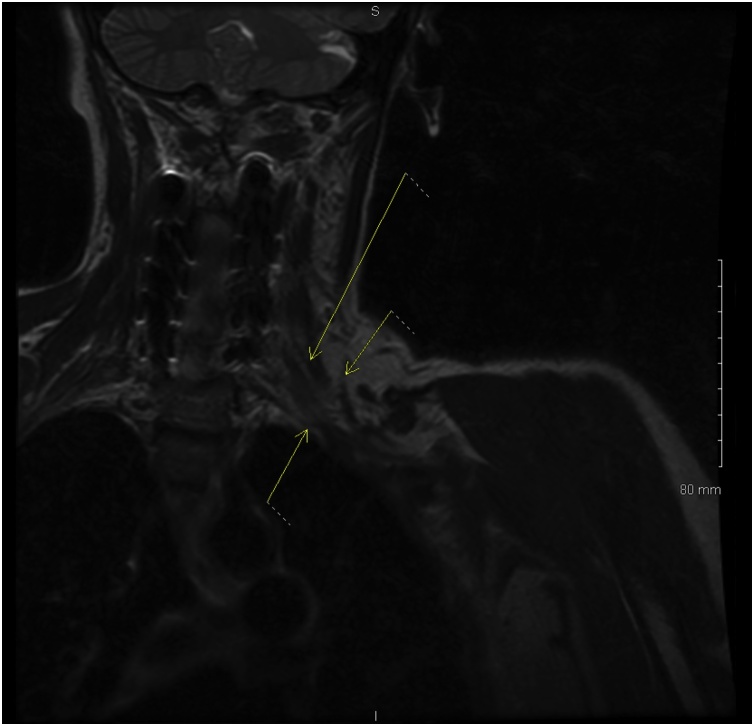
Postoperative onset of Brachial Neuritis shown through MRI.
